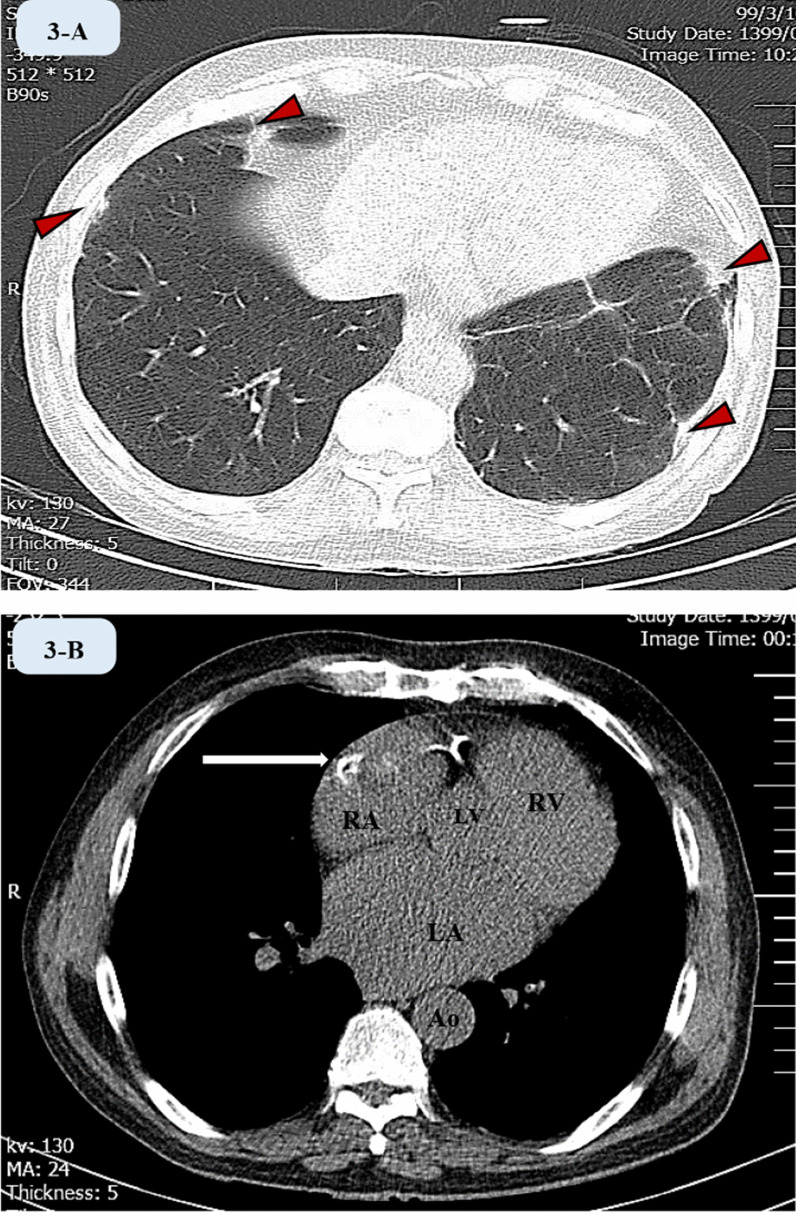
Computed tomography images.A Cross-sectional image of parenchymal window of the lungs showing bilateral fibrosis and mosaic pattern in both lungs with peripheral patchy ill-defined ground glass densities in favour of COVID-19 pneumonia (depicted via arrowheads). B Cross-sectional CT scan of the heart and mediastinum at the level of atria showing biatrial enlargement and a calcified space-occupying mass in RA (arrow). CT: computed tomography, LV: left ventricle, RV: right ventricle, LA: left atrium, RA: right atrium, Ao: descending aorta
